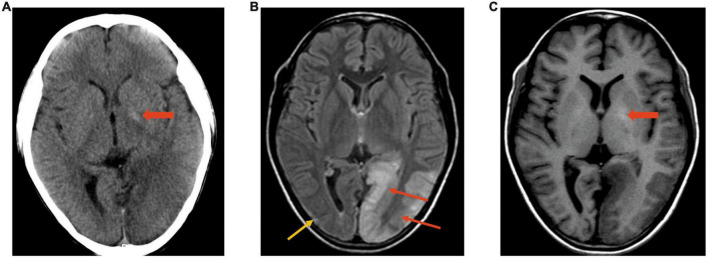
Polymorphism of lesions. Case 5, A 12-year-old boy with MELAS. (A) CT image. Density decreases in the left occipital lobe; calcification is slightly evident on the left (wide arrow). (B,C) MRI was performed after 3 days in the same boy. FLAIR (B) showed polymorphism of the lesion: Cortex swelling on the left occipital (red arrows) and cortex atrophy with gliosis (yellow arrow) on the right occipital. T1WI (C) showed hyperintensity in the bilateral basal ganglia and hypointensity in the left occipital region.
